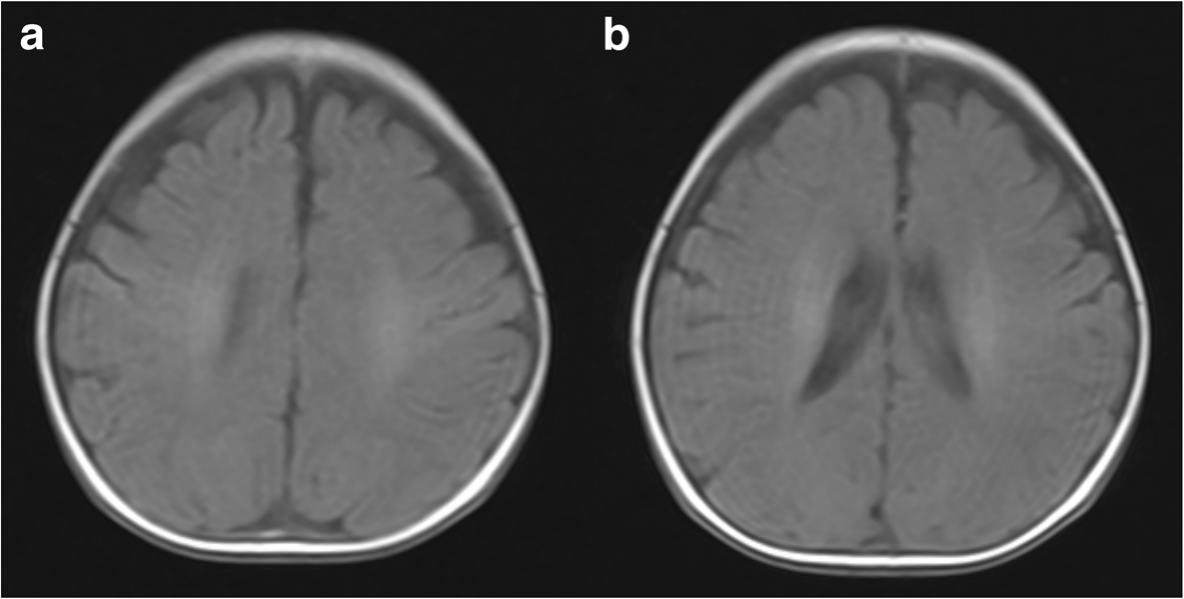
Head MRI of T1 WI axial images of life in the three-month-old. a and b demonstrate normal signals in centrum semiovale and periventricular white matter
